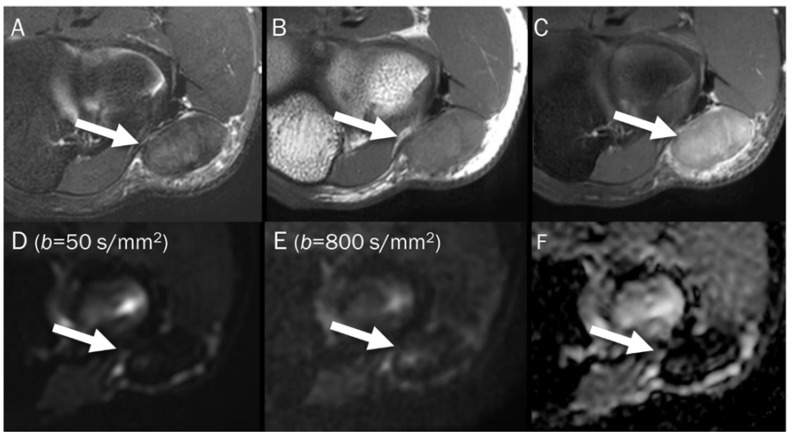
A 46-year-old male with a hematoma on his elbow caused by being struck with a baseball three months ago. Conventional axial MRIs (A: T2-weighted, B: T1-weighted, C: T1-weighted enhanced) show a well-circumscribed mass (arrow) with T2 high and T1 intermediate signal intensity, and heterogeneous enhancement in the elbow. (D,E) DWI with *b*-values of 50 and 800 s/mm^2^ exhibit persistent low signal (arrow) with different *b*-values, while (F) the ADC map shows a signal drop (arrow), indicating T2 “black-out” effect, possibly due to susceptibility artifacts.
